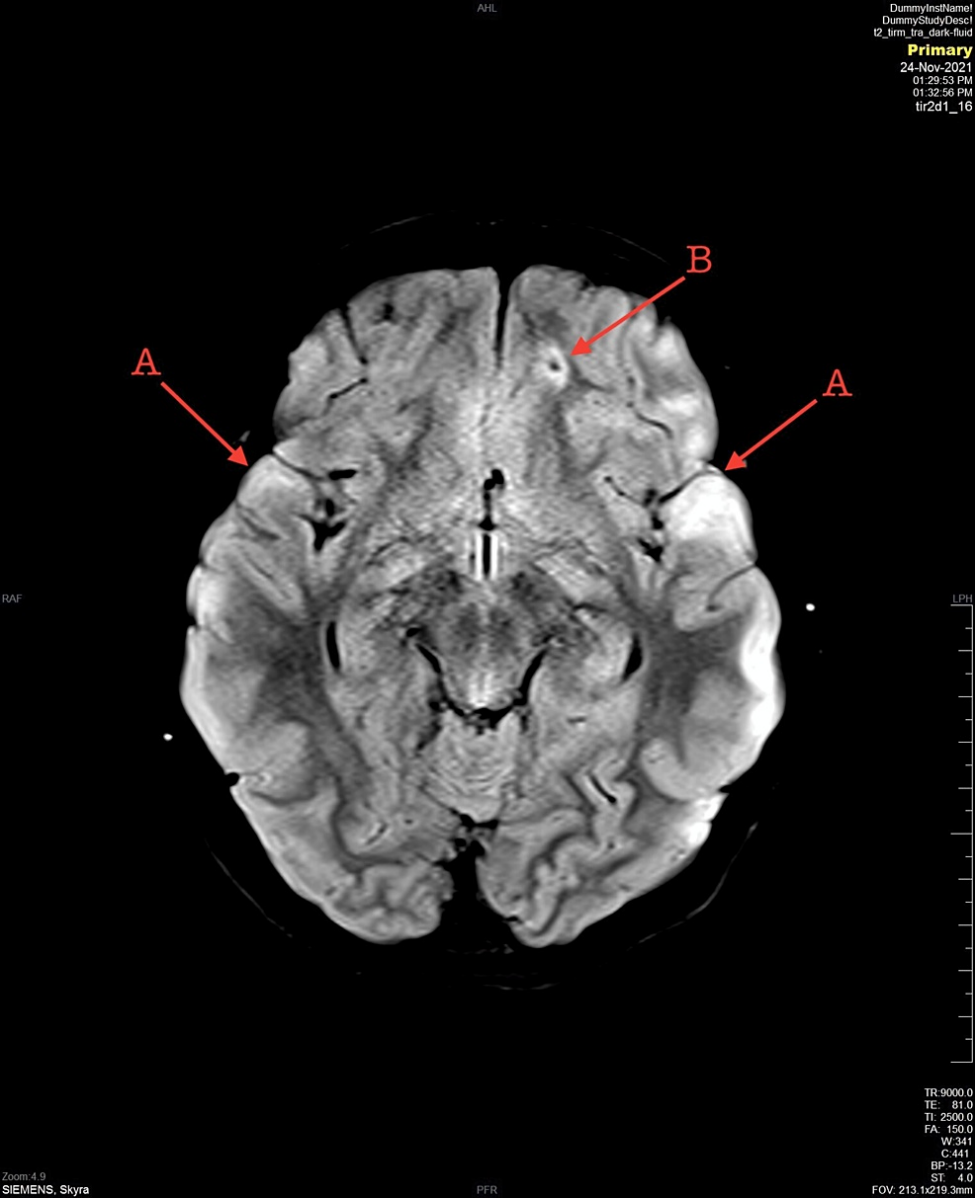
Bilateral hyperintense foci in the cortical (A) and subcortical (B) junction in fluid attenuated inversion recovery (FLAIR) MRI
